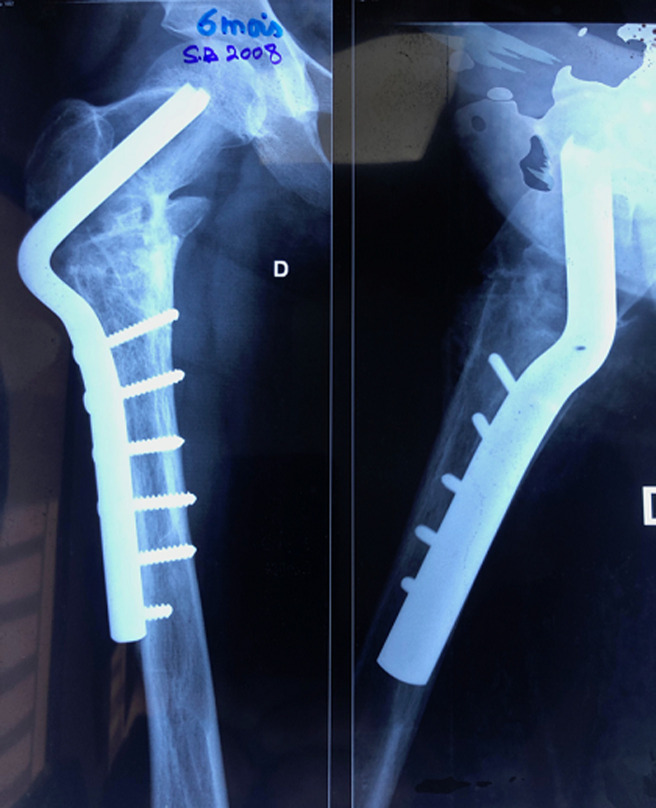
image radiographique à 6 mois de la mise en place d´une lame plaque
